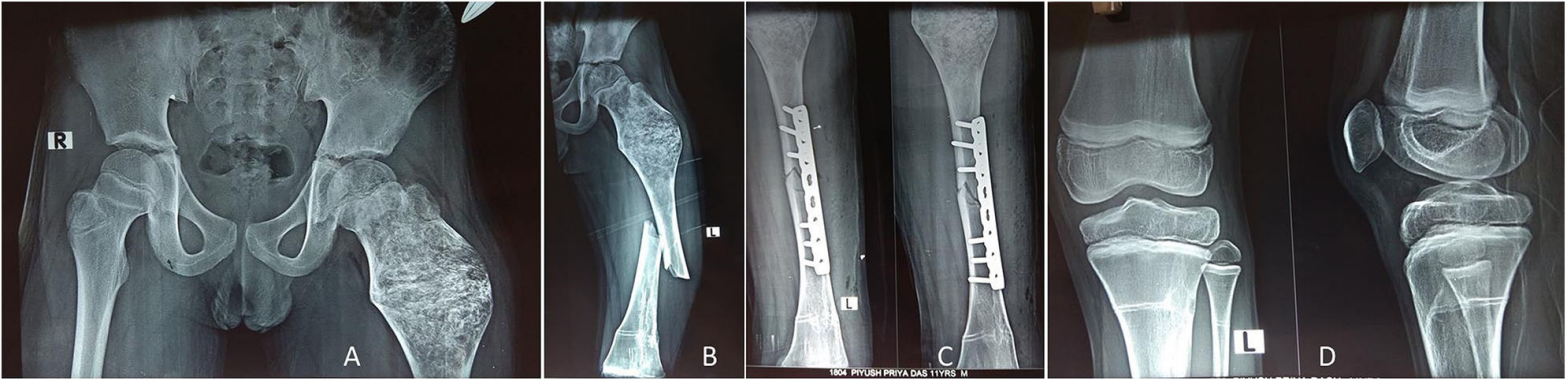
The child in Figure 6 received IV zoledronic acid infusion at every 6-month interval until 2 years, and the lesion healed up with mineralization (A). Four years later, the child presented with femoral shaft fracture (but not in the lesion area) after a fall while playing football (B), and it was treated with open reduction internal fixation with a plate (C). The X-ray around the knee showed multiple Harris growth arrest lines (zebra stripe sign) (D).
